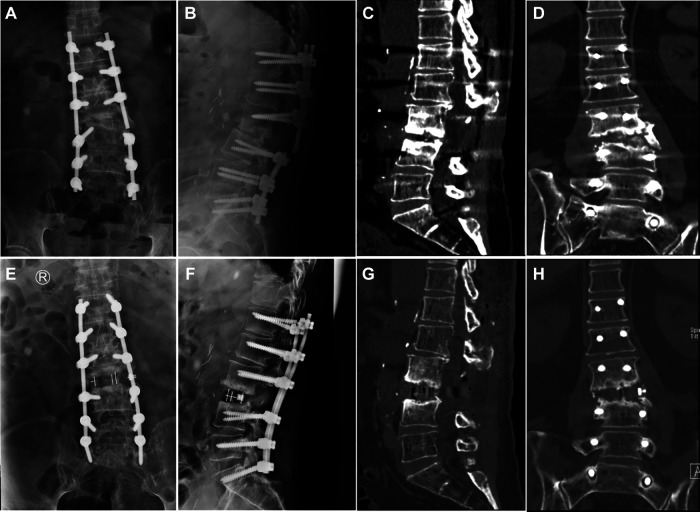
Preoperative frontal and lateral x-rays and CT showed bone resorption with bilateral rod fractures and pseudarthrosis formation (A–D). Postoperative images show a satisfactory reconstruction of lumbar curvature and height (E–G). A restored coronal balance (H).
